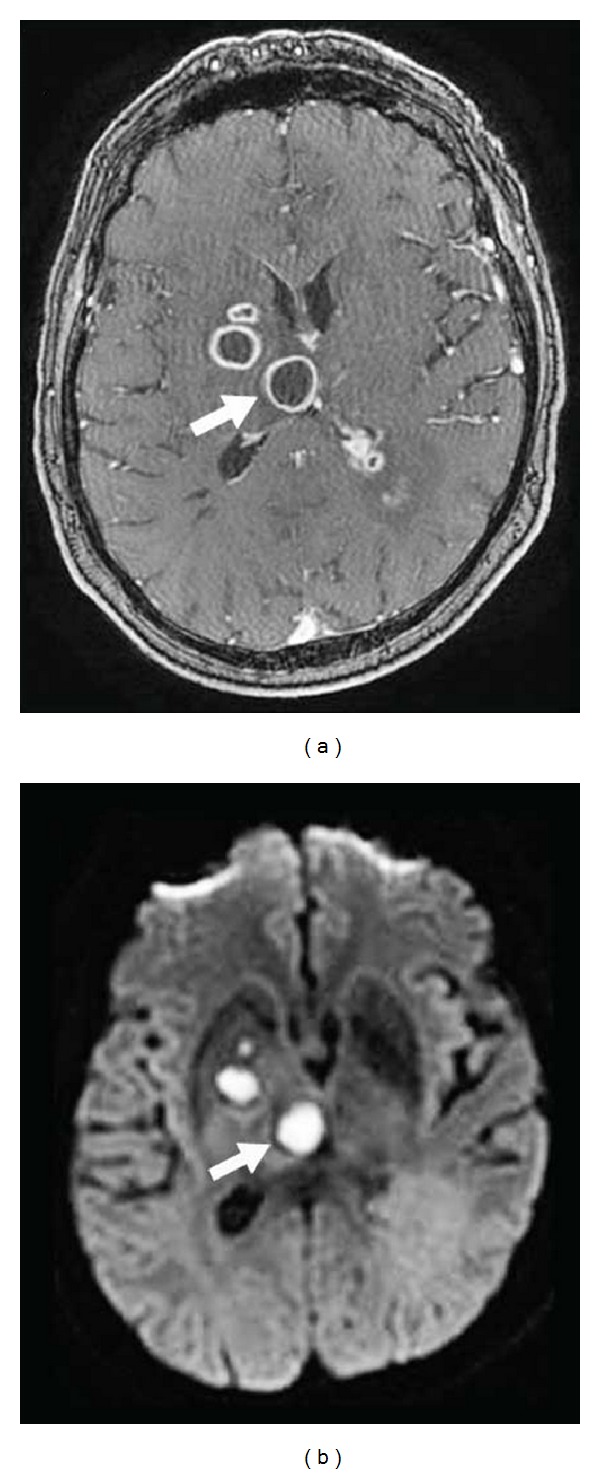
A patient with several brain abscesses involving the right thalamus (arrows) and the basal ganglia, with ring enhancement on gadolinium-enhanced T1-weighted imaging (a) and restricted diffusion seen as hyperintensity on DWI imaging (b).
